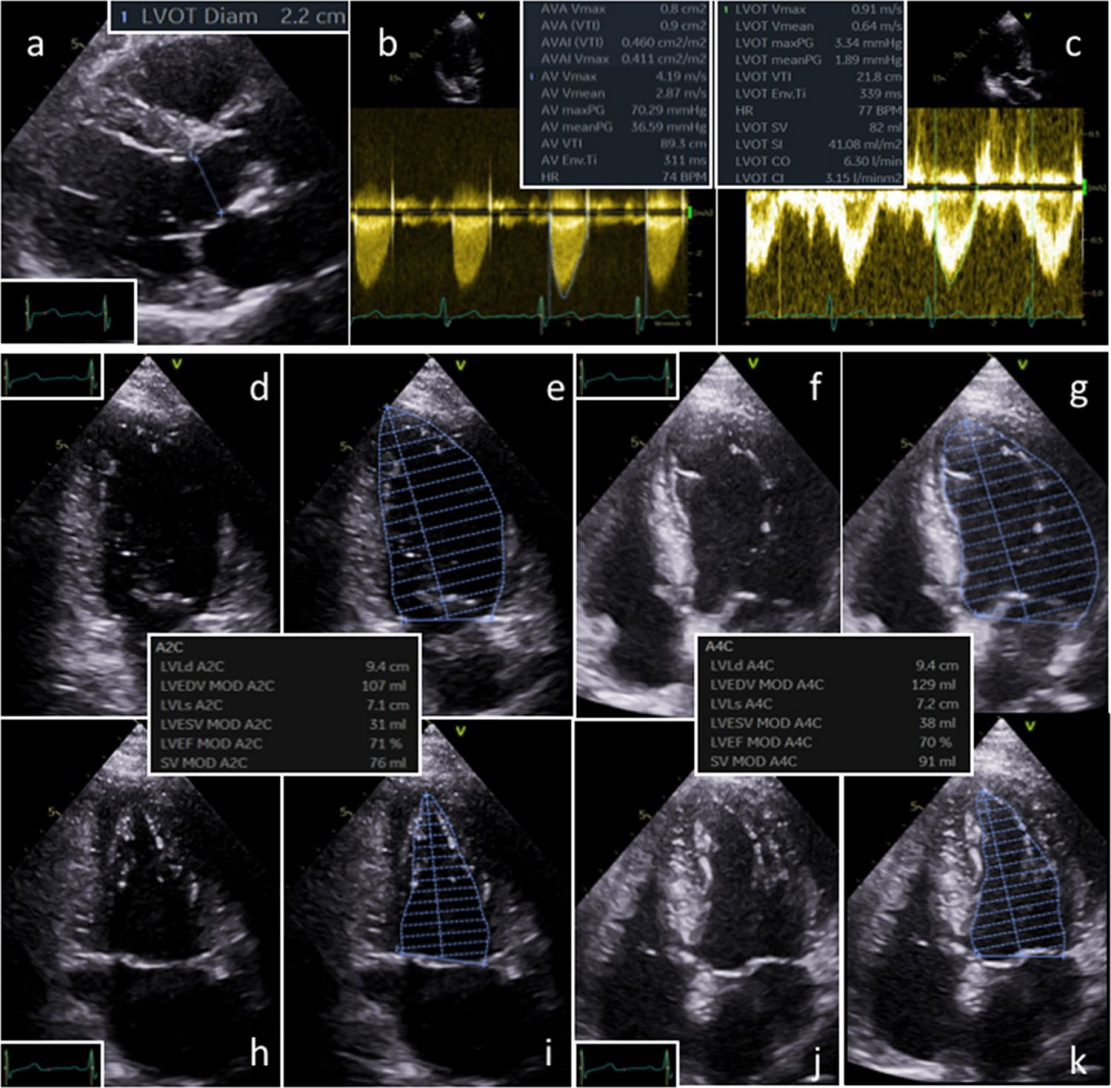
Assessment of effective Aortic orifice Valve Area (AVA) by continuity equation (a-c) and determination of left ventricular stroke volume (LVSV) by Doppler method (a,c) and by Simpson’s method (d-k). LV = left ventricle, LVOT = left ventricular outflow tract, 2C = 2-chamber, 4C = 4-chamber, SV = stroke volume, ESV = end-systolic volume, EDV = end-diastolic volume, EF = ejection fraction, Vmax = maximum flow velocity, maxPG = maximum pressure gradient, meanPG = mean pressure gradient VTI = velocity time integral, CO = cardiac output, HR = heart rate, CI = cardiac index, SI = stroke index
